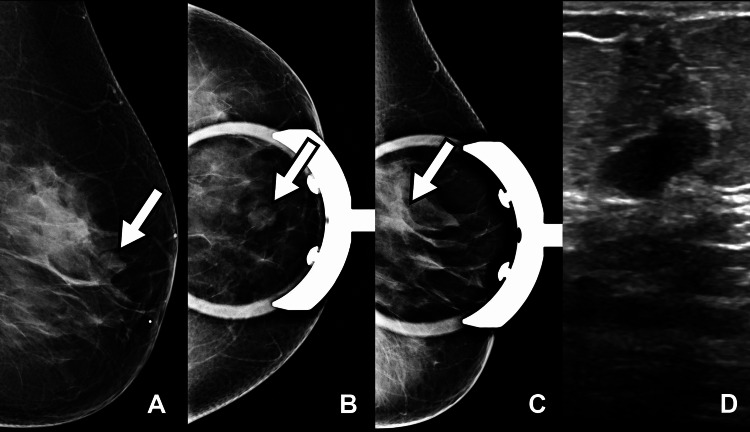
(A-C): Mammogram demonstrates an equal density oval mass with microlobulated margins measuring 11 mm x 11 mm x 5 mm in the upper inner quadrant at 11 o'clock, 2 cm from the nipple. (D): Ultrasound showed an 11 mm x 11 mm x 5 mm solid oval-shaped mass with microlobulated margins and non-parallel orientation in the upper inner quadrant at 11 o'clock, 2 cm from the nipple.
